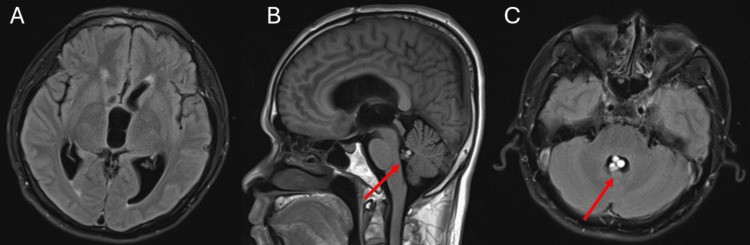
MRI of the brain on hospital day eight showing interval migration of cystic lesions from the third ventricle to the fourth ventricle. A: Axial T2 fluid-attenuated inversion recovery imaging shows persistent ventriculomegaly without evidence of the previously noted cyst in the third ventricle. B: Sagittal T1-weighted image shows ventricular dilation without evidence of the previously noted third ventricular cyst. C: Axial T1-weighted imaging shows a migrated cystic lesion now in the fourth ventricle.
